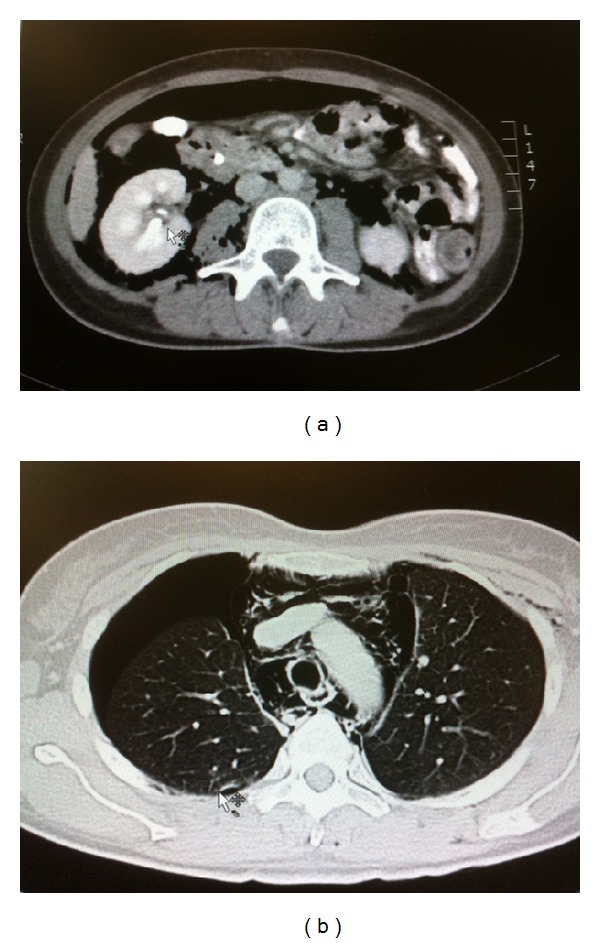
(a) Abdominal CTscan showing intra- and retroperitoneal free air. (b) Thoracic CT showing right-sided pneumothorax and presence of mediastinal air.
